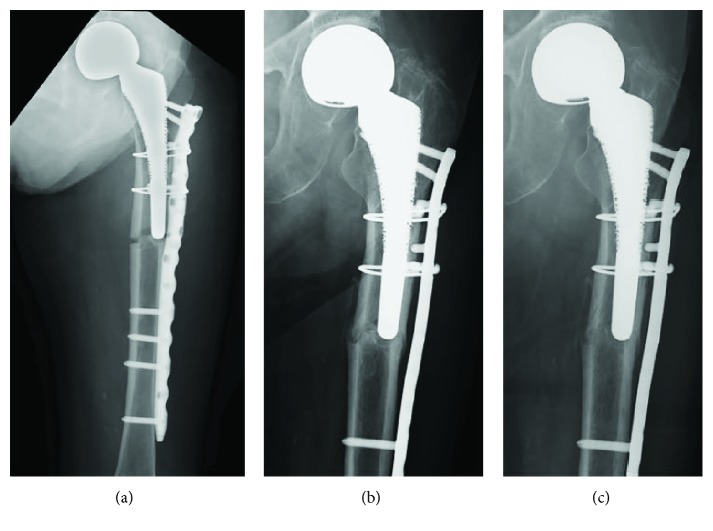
Anteroposterior radiographs showing fixation of the periprosthetic fracture using a cable-plate device (a) after the operation, (b) at one-year follow-up, and (c) at two-year follow-up.
